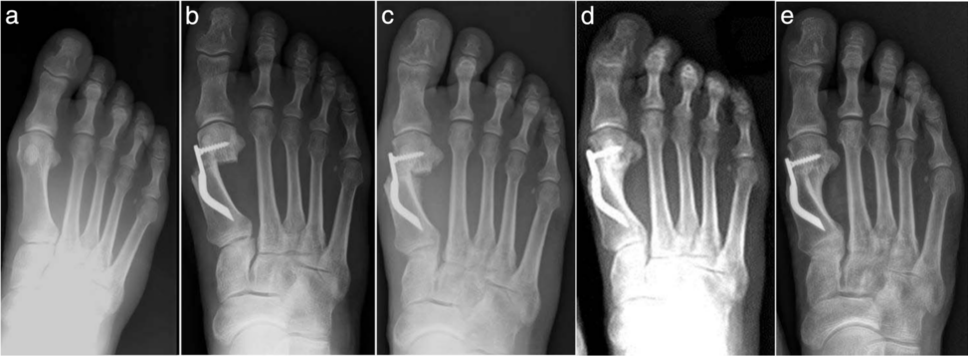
Case 8. A 38-year-old woman with mild HV; antero-posterior radiographic images at: a Preoperative period. b 1-month follow-up. c 3-month follow-up. d 6-month follow-up. e 48-month follow-up showing the maintained correction of the deformity
